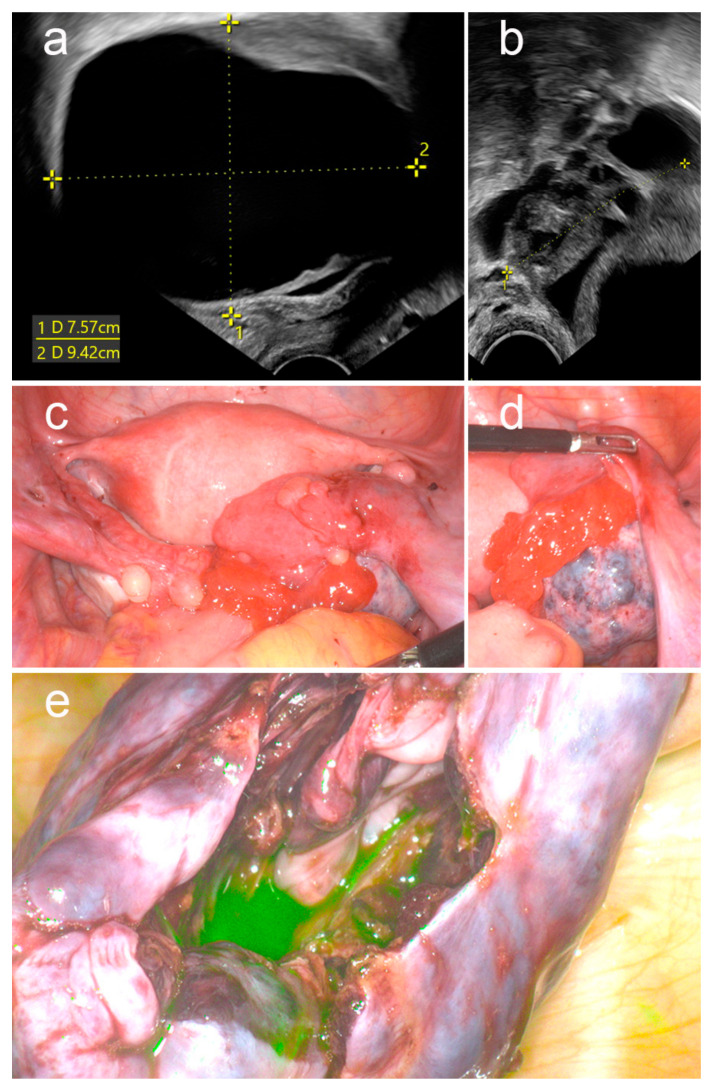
Ultrasonic (a,b) and intraoperative (c–e) presentation of the right ovary. The right ovary shows an ovarian cyst measuring 75.2 × 94.2 mm (a) with ovarian stromal edema and edema around the ovary (b). Intraoperatively, the right ovary appears lived and ischemic (c,d). Following detorsion and cystectomy, ICG angiography demonstrated restored ovarian perfusion (e). The photos were taken with the pinpoint endoscopic fluorescence imaging camera system (Stryker^®^, Duisburg, Germany).
